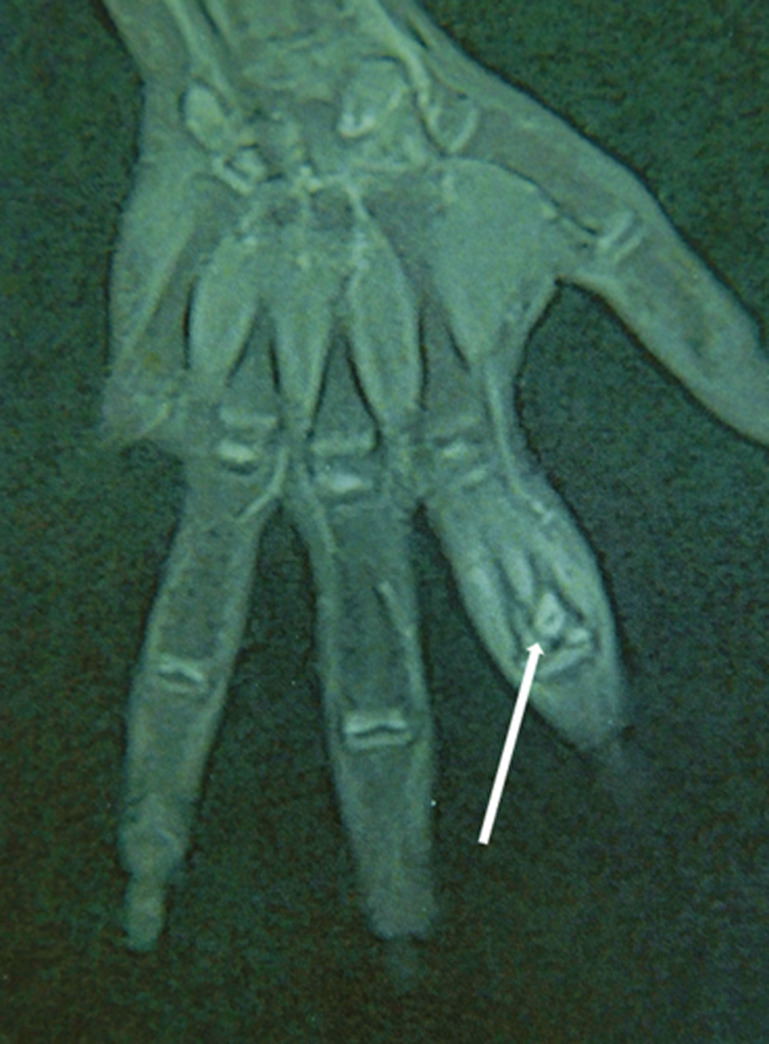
magnetic resonance imaging coronal image of the hand revealing a well-defined corticomedullary lesion in the metaphyseal-epiphyseal region with a punctate osseous center, cortical erosion at the articular surface, and associated bone marrow edema (white arrow)
